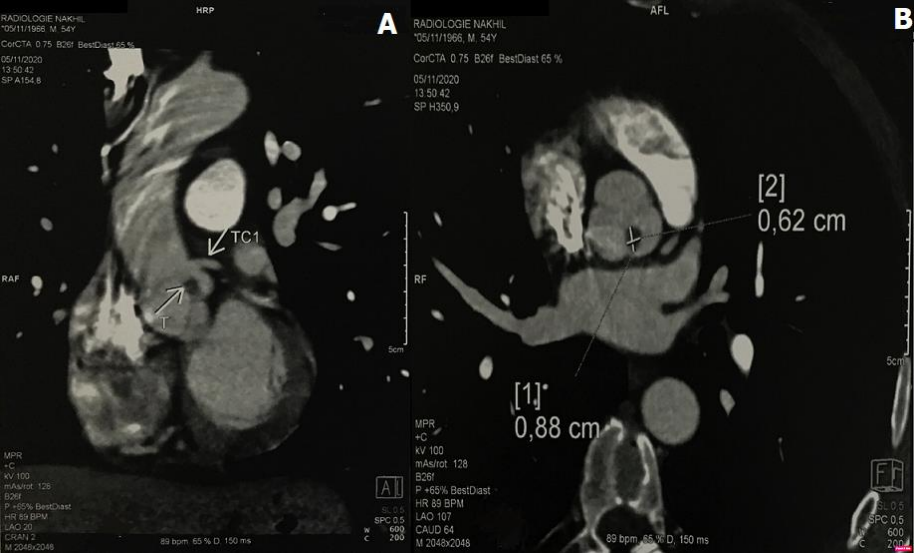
computed tomography angiography shows a 0.62/0.88 cm mass attached to aortic leaflet, near the ostium of the main left coronary artery (A, B)
